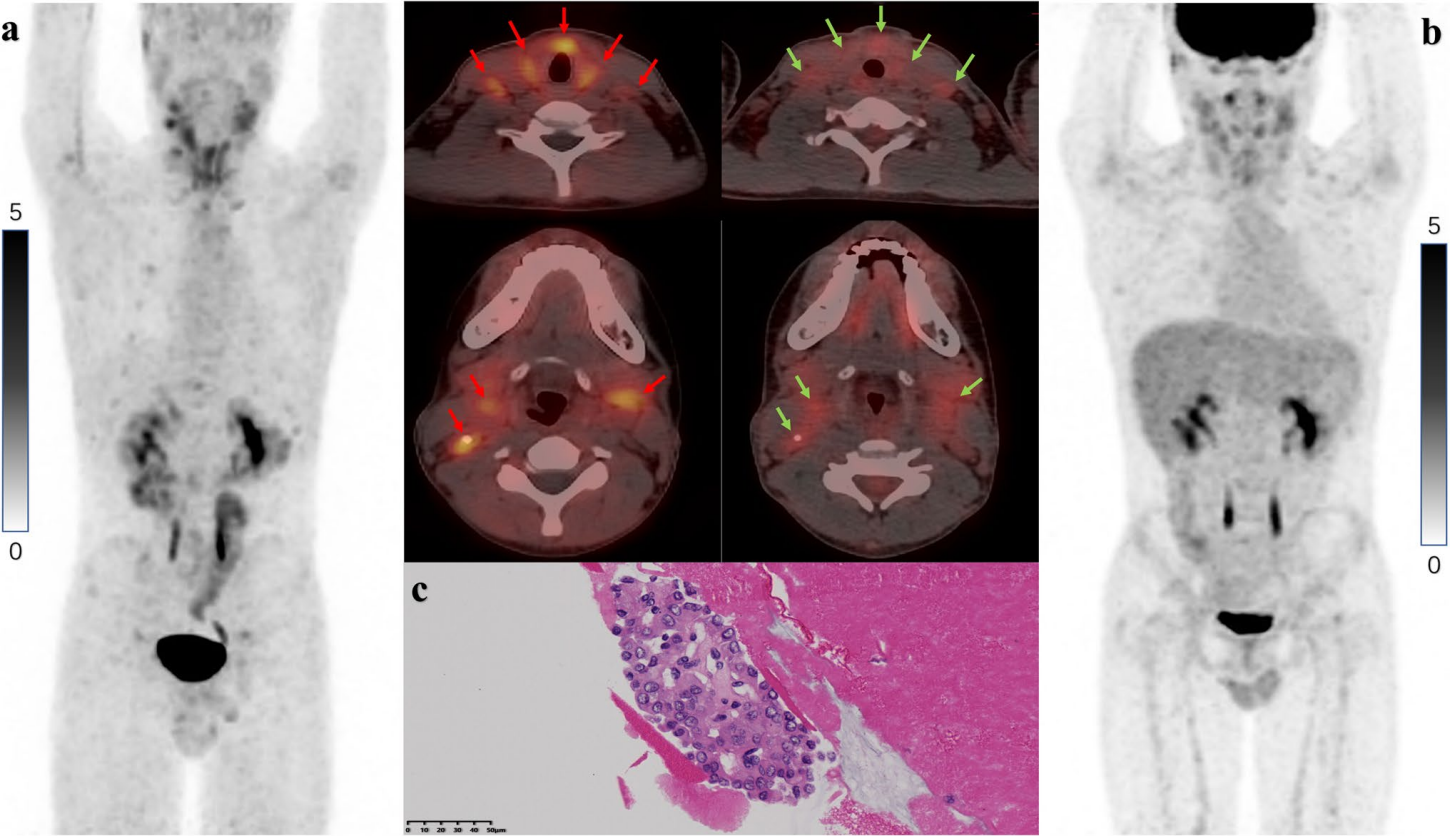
Local recurrences and lymphatic lesions in a 12-year-old boy (only this patient is younger than 18). a [^18^F]FAPI-42 PET/CT revealed local recurrence and lymphatic lesions with moderate-to-intense [^18^F]FAPI-42 uptake (lesions are indicated by red arrows on axial fusion images). b The uptake of 2-[^18^F]FDG was lower than that of [^18^F]FAPI-42 (lesions are indicated by green arrows on axial fusion images). c Pathologically confirmed diagnosis of PTC via US-FNA
